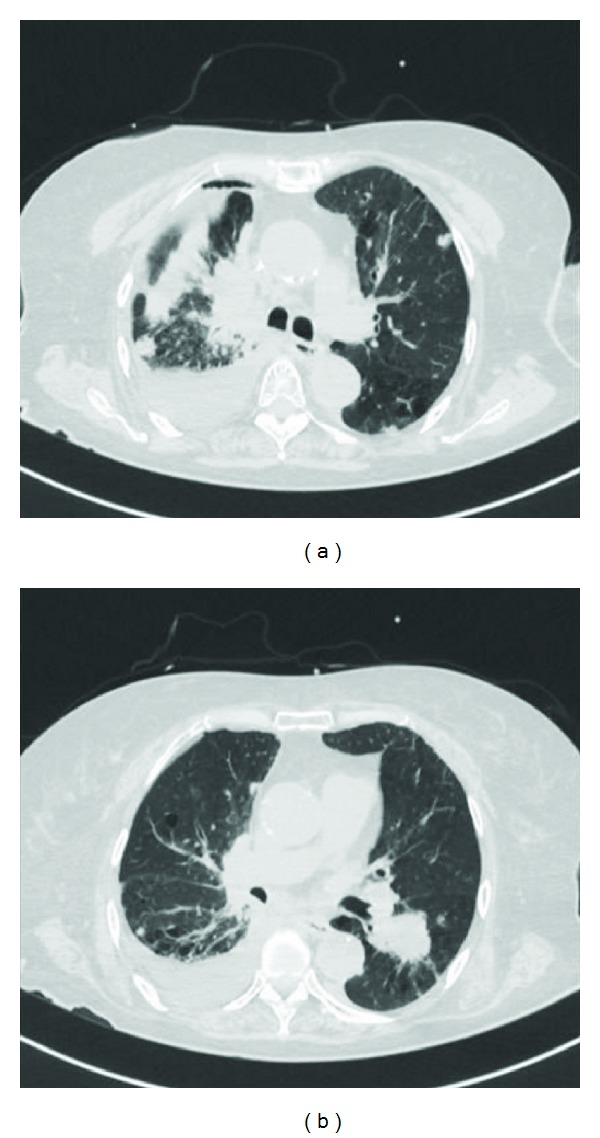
Chest CT scan demonstrated multiple tumors in the bilateral lungs and a right-sided pleural effusion.
